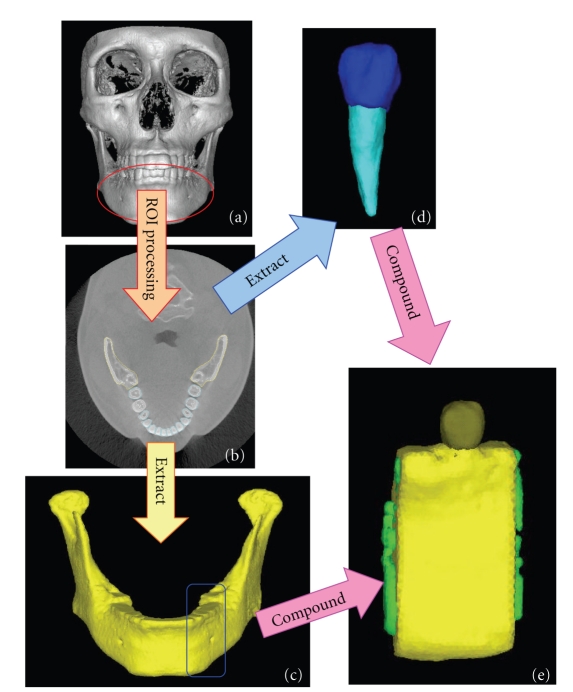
Flow of procedure for the FE modeling of the mandibular bone and tooth by MF. (a) CT scan of the testee, (b) extracting bone lines, (c) mandibular bone, (d) tooth, (e) 3D FE models.
